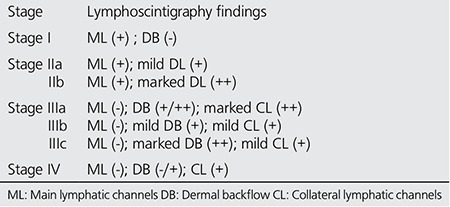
Lymphoscintigraphic staging (by Lee and Bergan)
